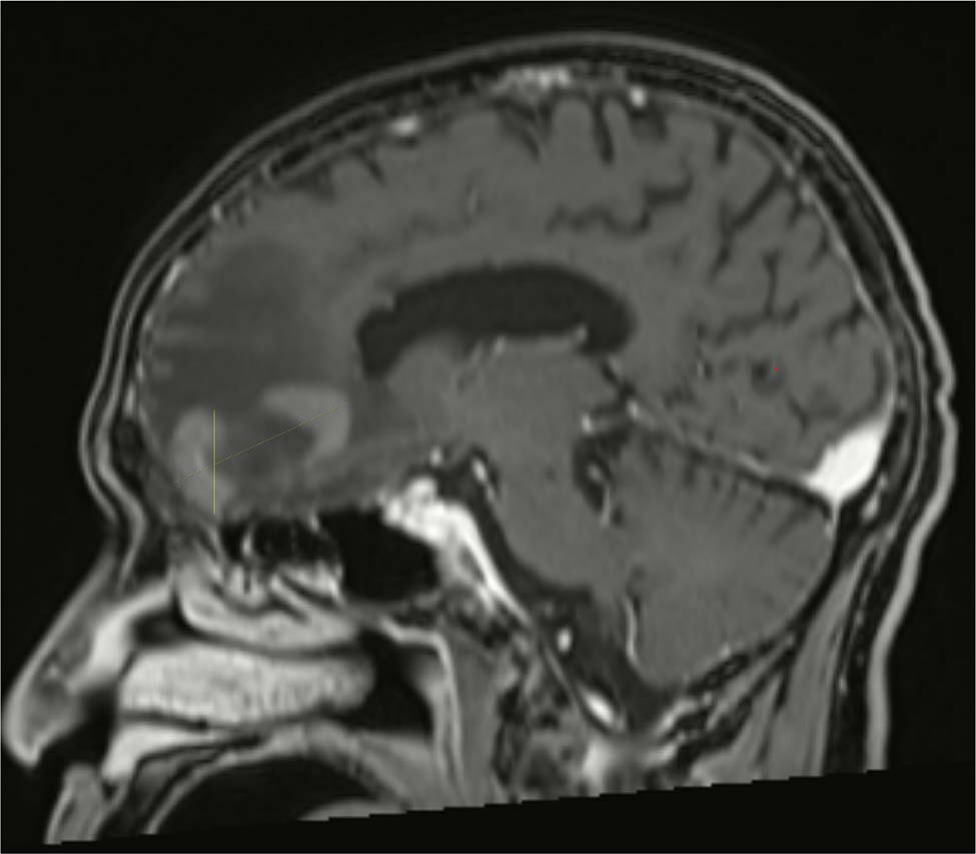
MRI brain showing right frontal lobe mass.
